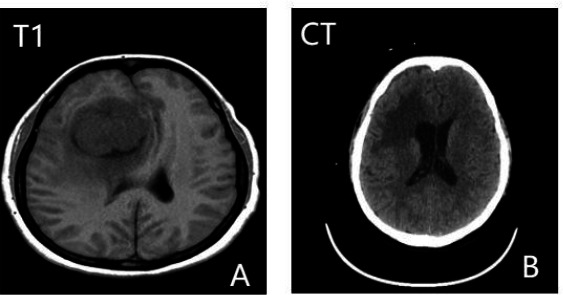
(A) Axial view of the preoperative T1-weighted MRI; (B) Axial view of the CT scan performed at discharge.
